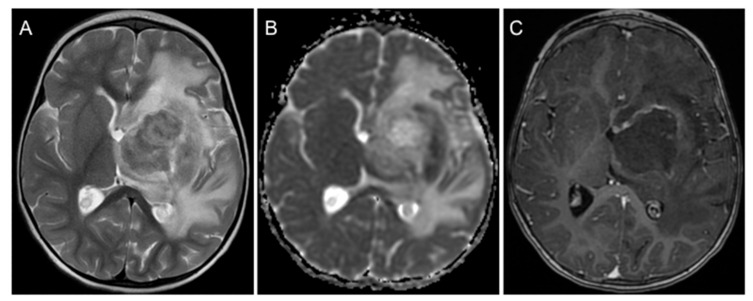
Imaging features of primary central nervous system lymphoma. (A) An axial T2-weighted magnetic resonance image shows a large left-sided mass primarily located in the basal ganglia/thalamus with considerable perifocal edema. (B) Diffusion-weighted imaging (apparent diffusion coefficient map) demonstrates diffusion restriction in the periphery of the tumor, indicating high cellularity. (C) Following intravenous contrast medium administration, relatively little inhomogeneous enhancement is seen at the margins of the mass.
